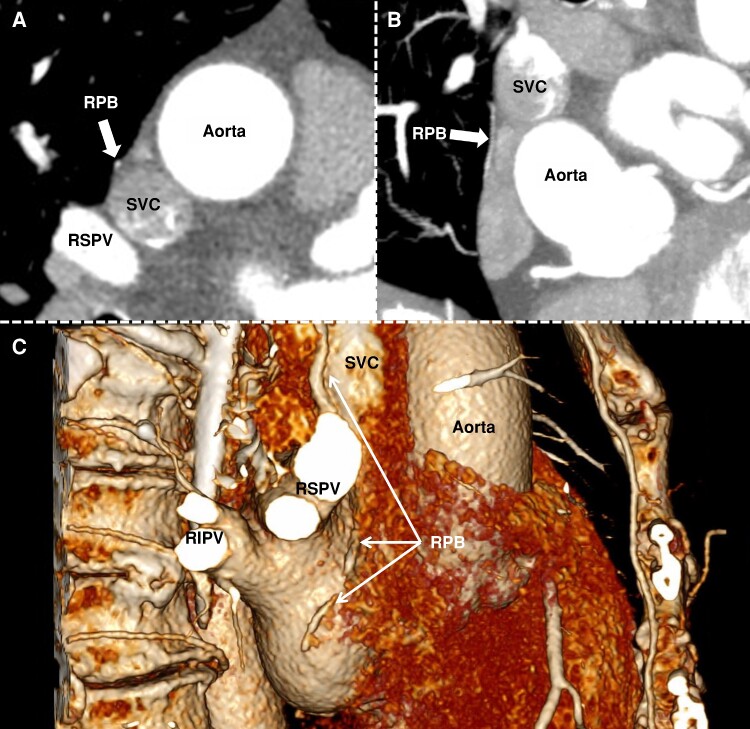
Delineation of the pericardiac RPB course using CT scan. *Panel A* shows an axial slice at the level of the RSPV, and *Panel B* an adjusted coronal slice, with the RPB highlighted (white arrow). *Panel C* shows a right lateral view of the 3D reconstruction of the CT scan, with the pericardiac course of the RPB highlighted (white arrows). RIPV, right inferior pulmonary vein; RPB, right pericardiophrenic bundle; RSPV, right superior pulmonary vein; SVC, superior vena cava.
